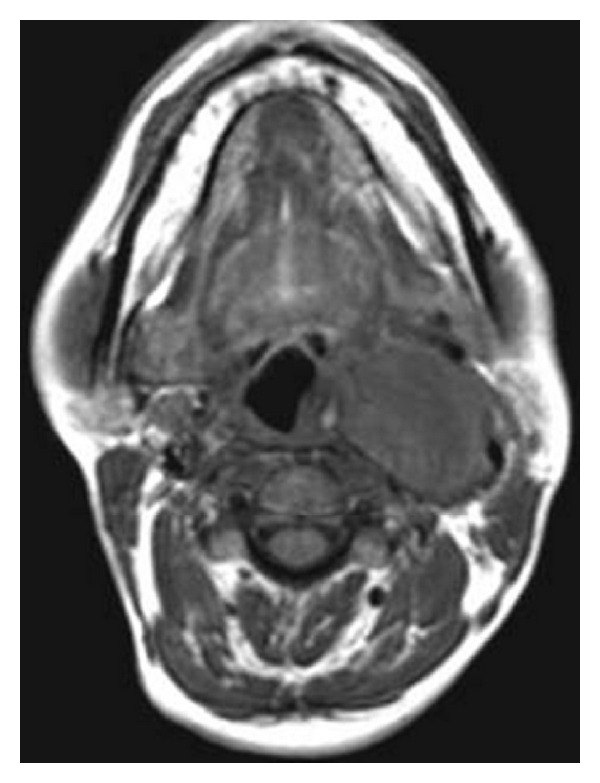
T1-weighted MR image reveals isointense mass on the left carotid space.
